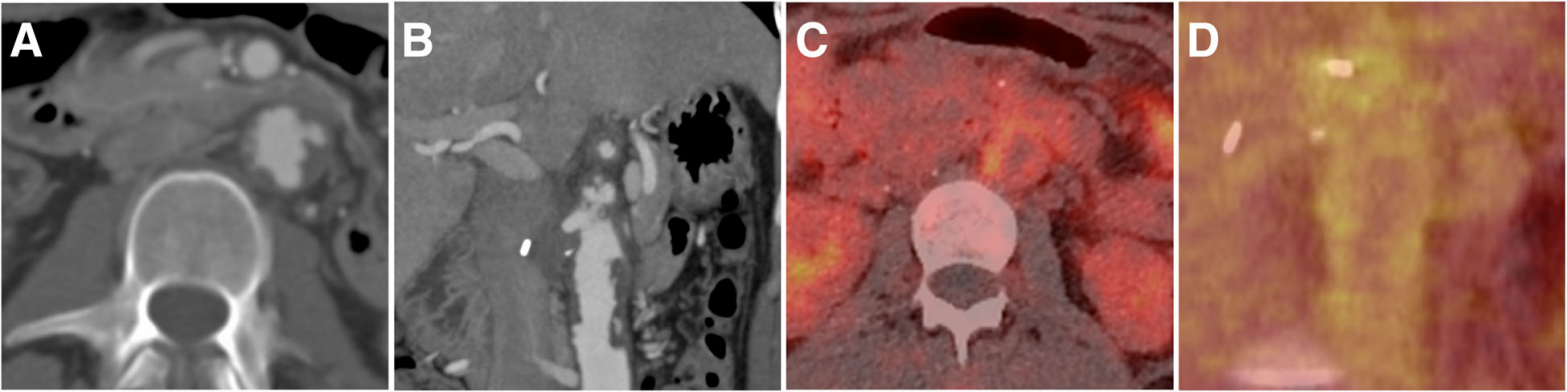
Angiosarcoma of the abdominal aorta in a 22-year-old woman. Computed tomography angiography (CTA) depicts tumorous mass extending within the aortic lumen resembling thrombus or atheroma (a, b). The intraluminal mass however showed increased metabolic activity on ^18^F- FDG-PET/CT (c, d), indicative of a malignant tumor
